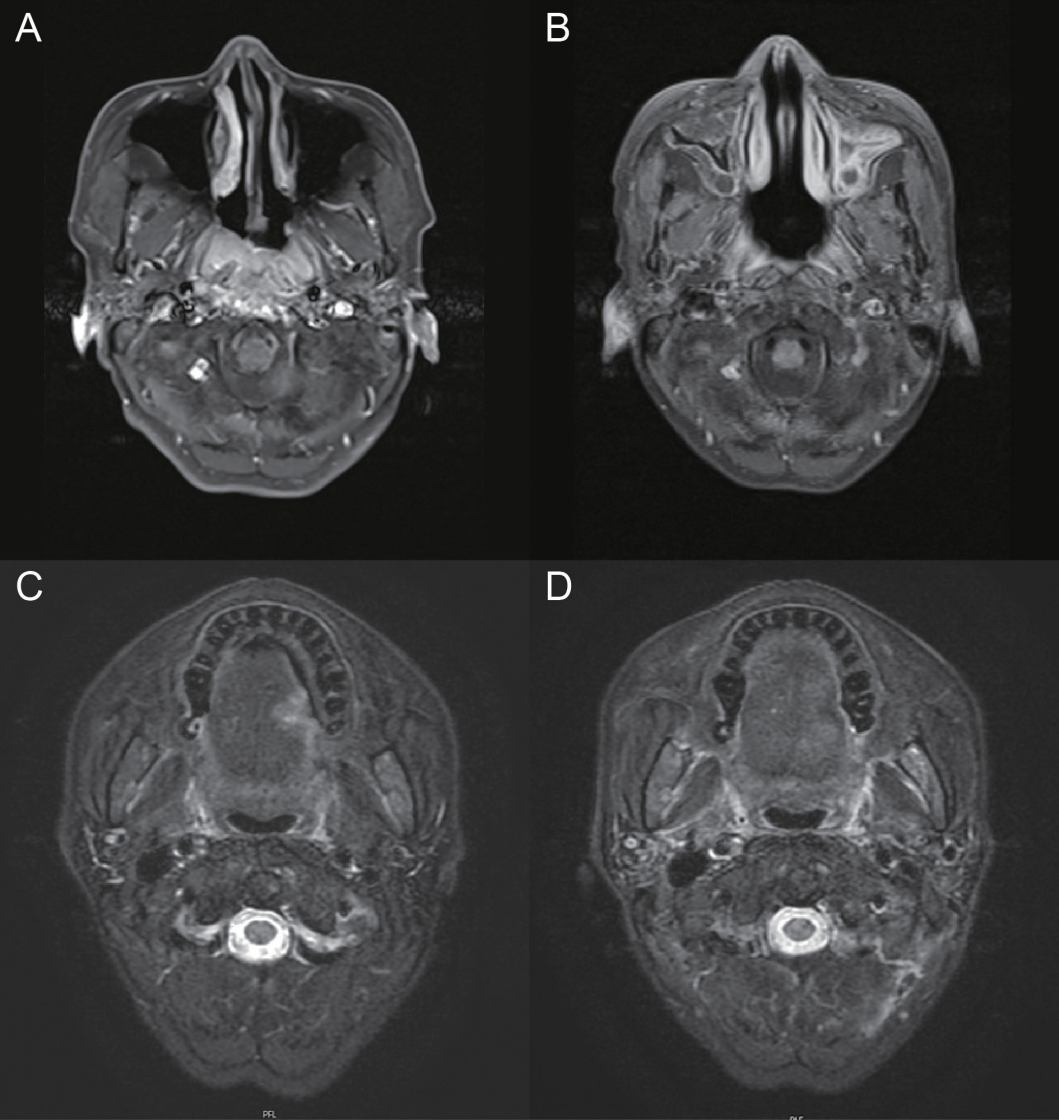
Pre- and post-treatment MRI of nasopharyngeal carcinoma and tongue cancer. (A) MRI depicting the nasopharyngeal lesion. (B) MRI showing the response of nasopharyngeal carcinoma after treatment. (C) MRI depicting the left tongue lesion. (D) MRI showing the response of tongue cancer after radiotherapy.
